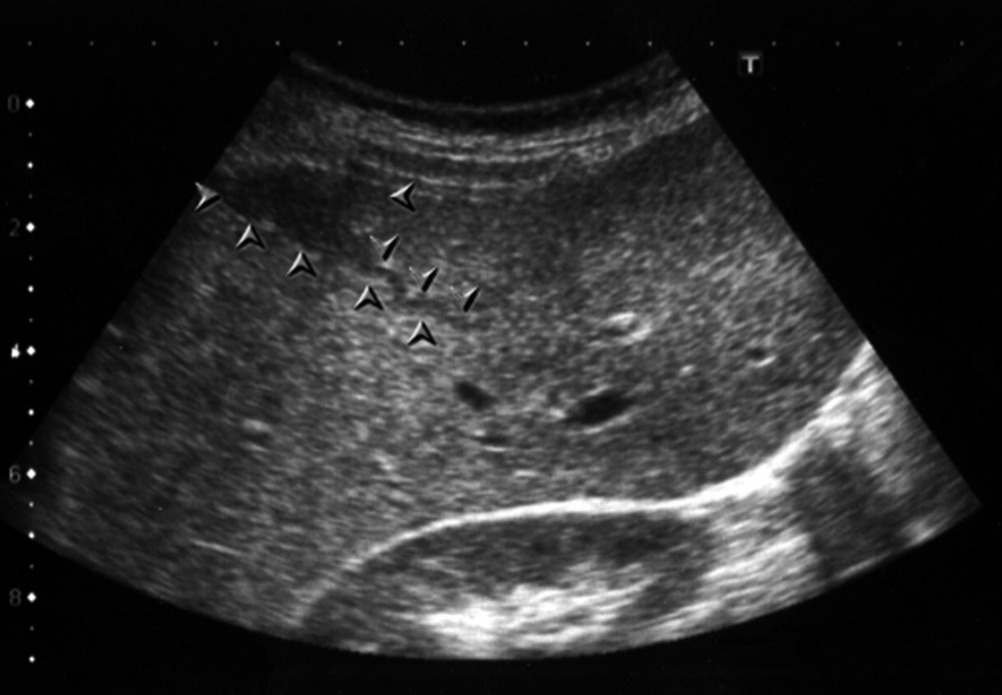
Ultrasound image of the liver reveals poorly defined hypoechoic subcapsular area and a tortuous tract, extending from the capsule to the deeper parenchyma (arrowheads).
